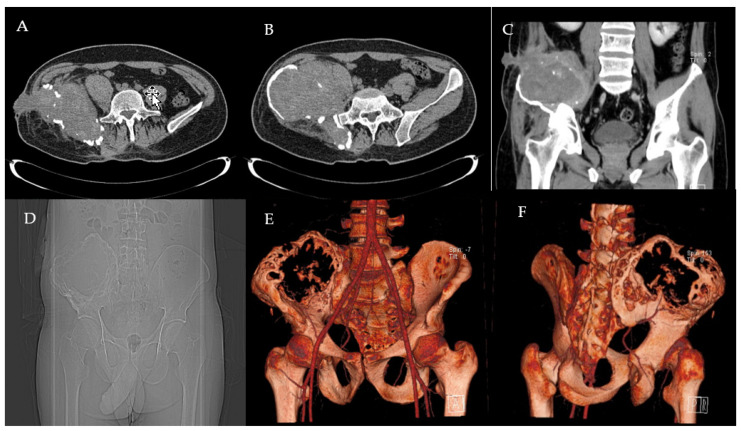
Preoperative CT scans (A–C), radiograph (D) and 3D reconstruction (E,F) of the pelvis with the right iliac pseudotumour.
